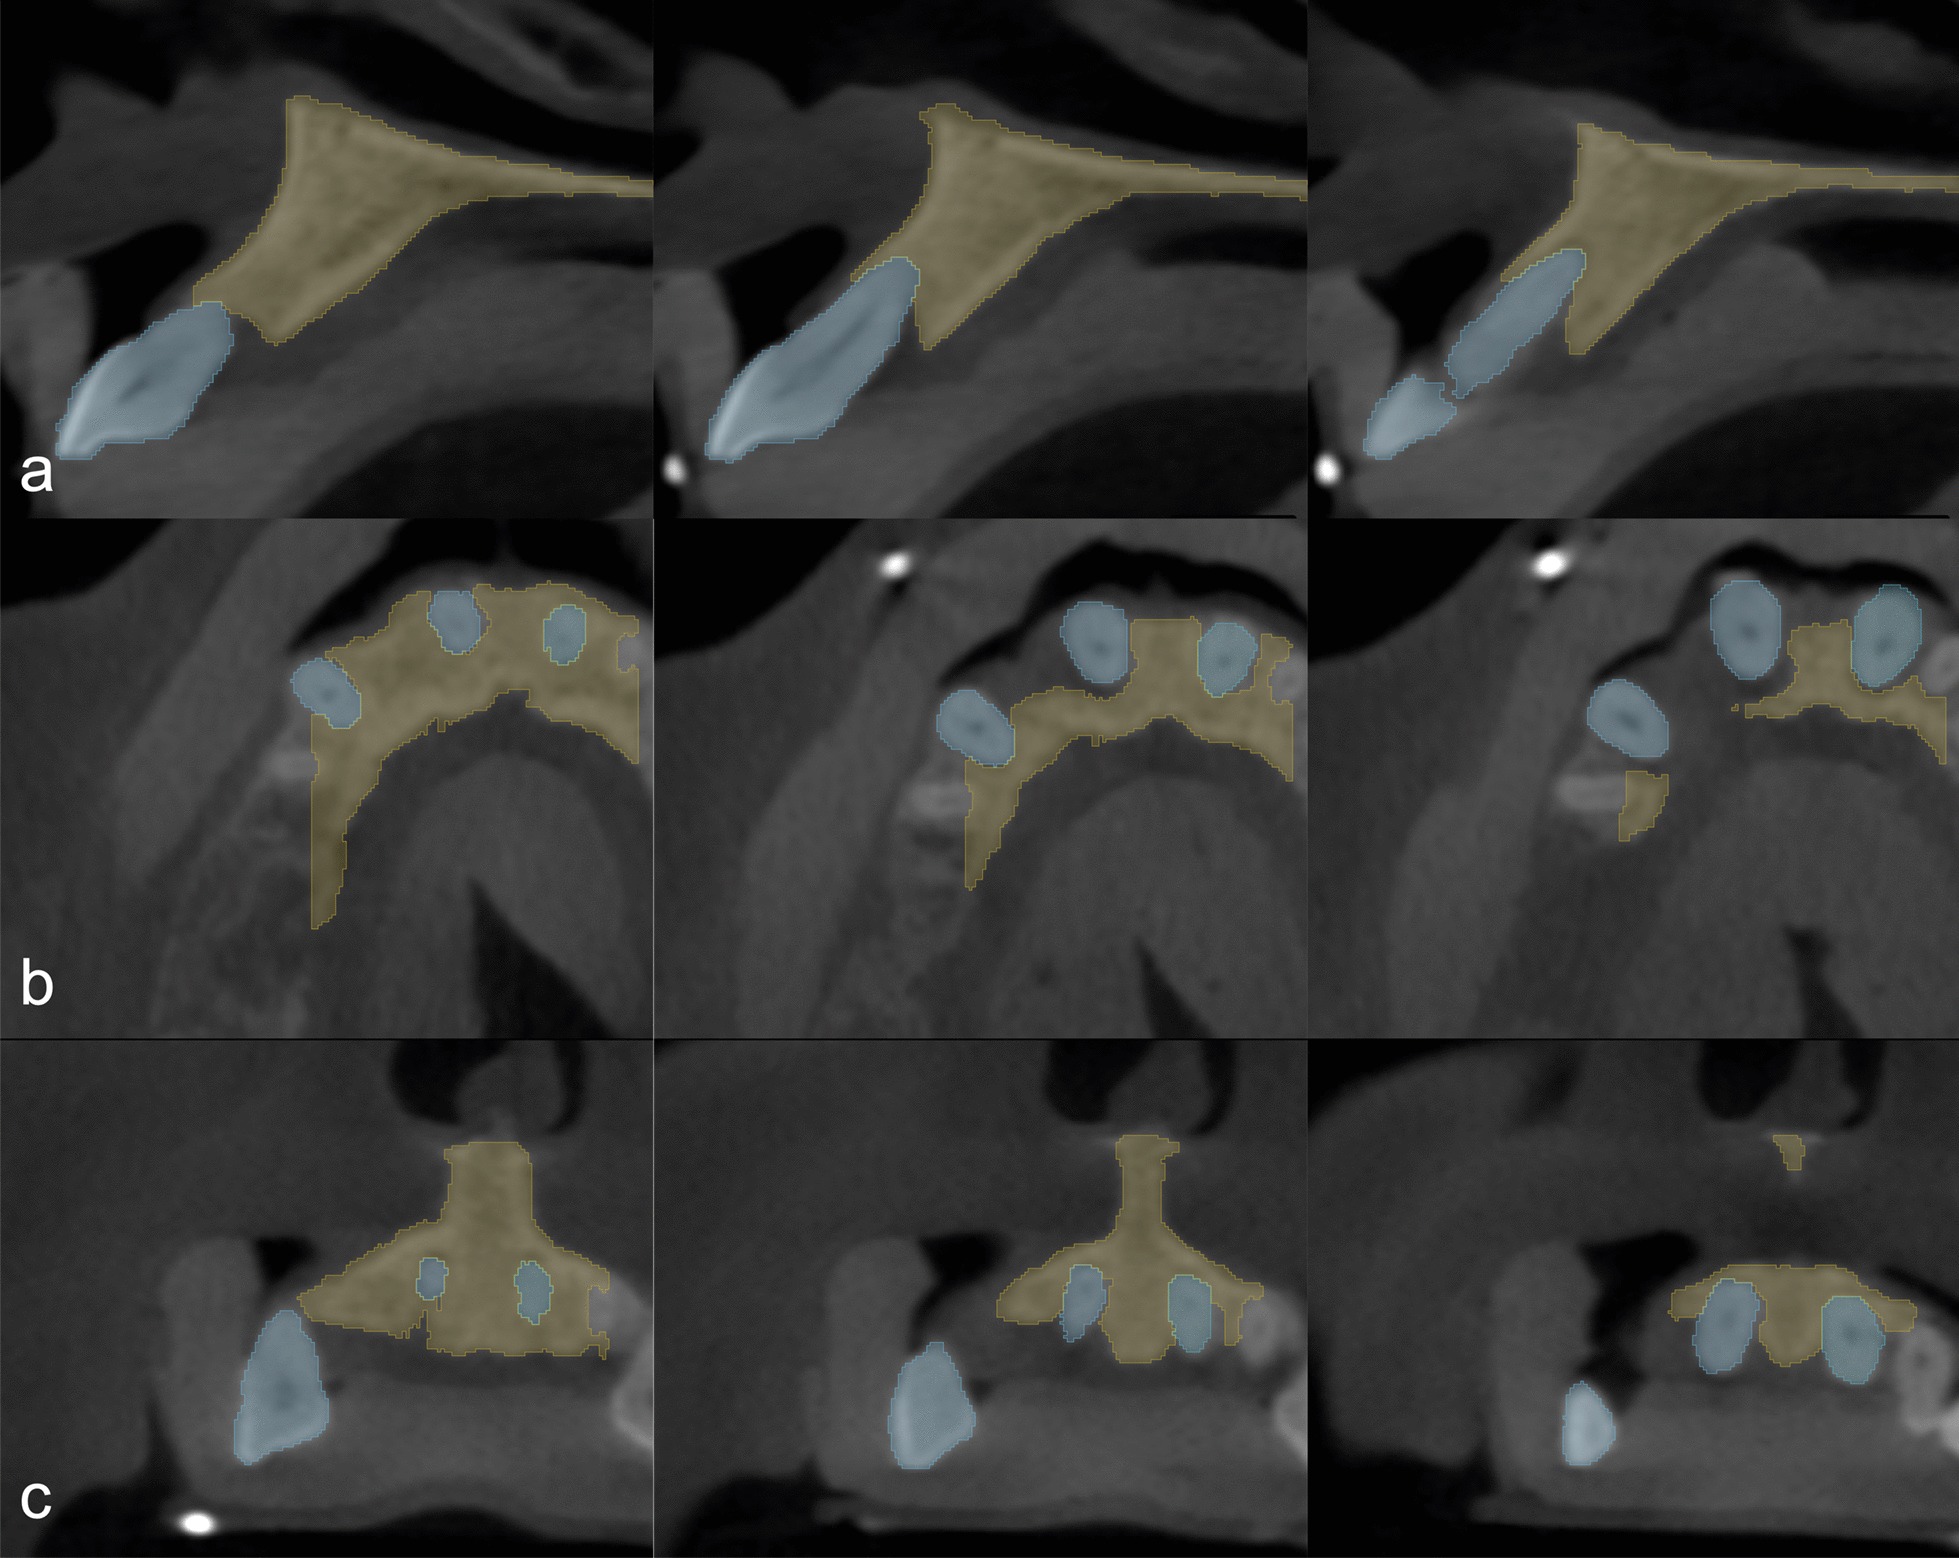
Regions of interest highlighted on slices of the CBCT dataset (brown: alveolar bone; blue: teeth). a Sagittal view. b Axial view. c Coronal view
